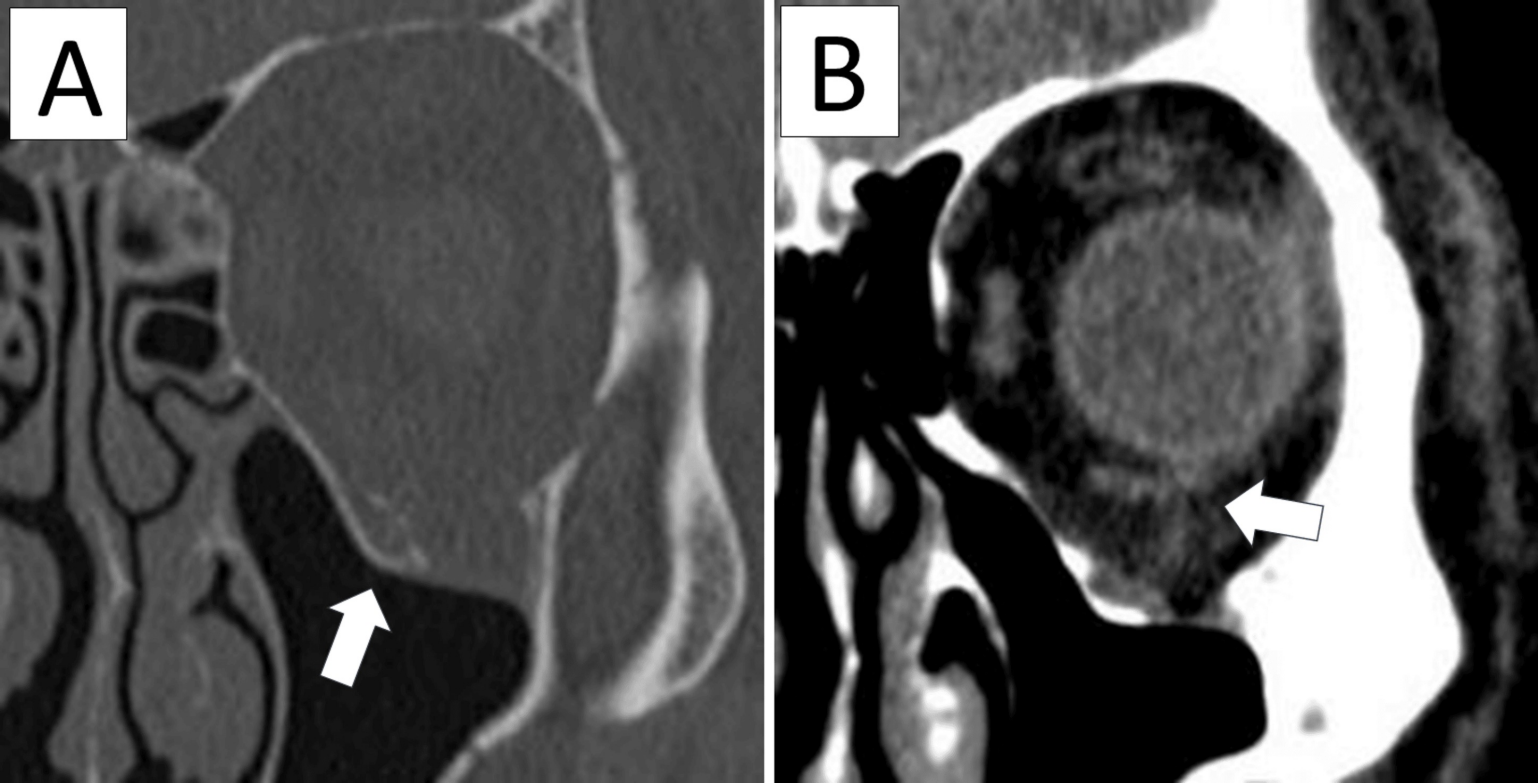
CT at five months post-injury The orbital floor bone (arrow) has mostly healed in a displaced position. The inferior rectus muscle (arrow) has returned to its normal location.
